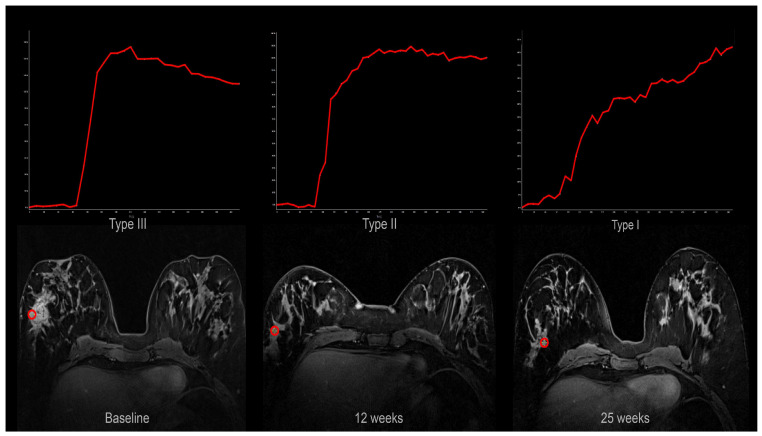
Signal intensity–time curves from one patient at baseline (type III; wash-out), 12 weeks (type II; plateau), and 25 weeks (type I; persistent). The curves were obtained from the region of interests (red circle) shown in the corresponding axial DCE MRI images.
